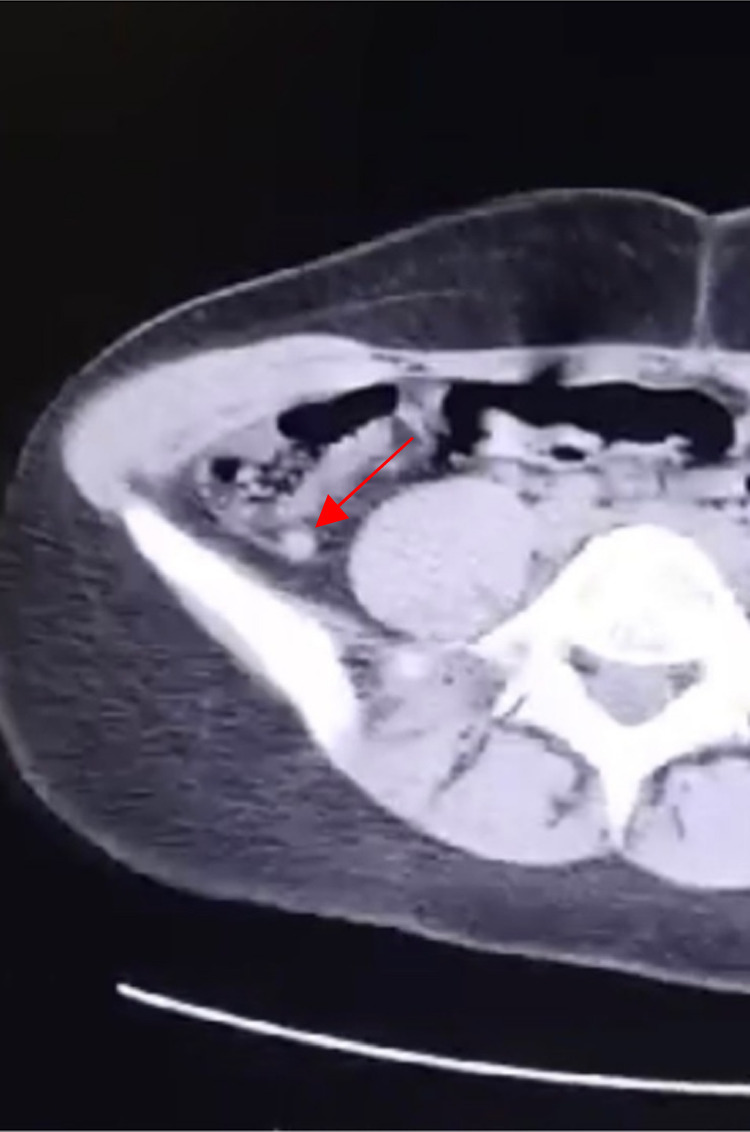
Computed tomography scan showing early signs of appendicular inflammation, arrow above.
